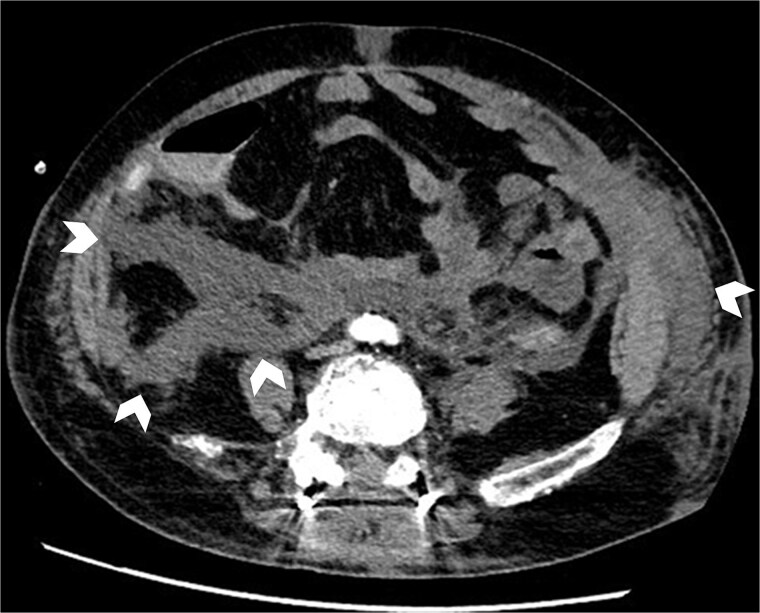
POD 5 CT showing progressive fluid collection in the retroperitoneal space (arrowheads).
